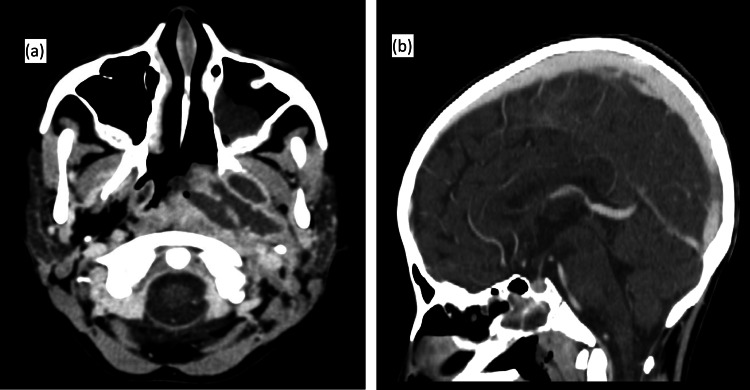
CT images of the patient after the first cycle of chemotherapy (a) Axial CT image at the level of the nasopharynx showing regression in size of the tumoral process along the posterior wall of the nasopharynx. (b) Sagittal CT image with contrast injection demonstrating good patency of the superior sagittal sinus.
